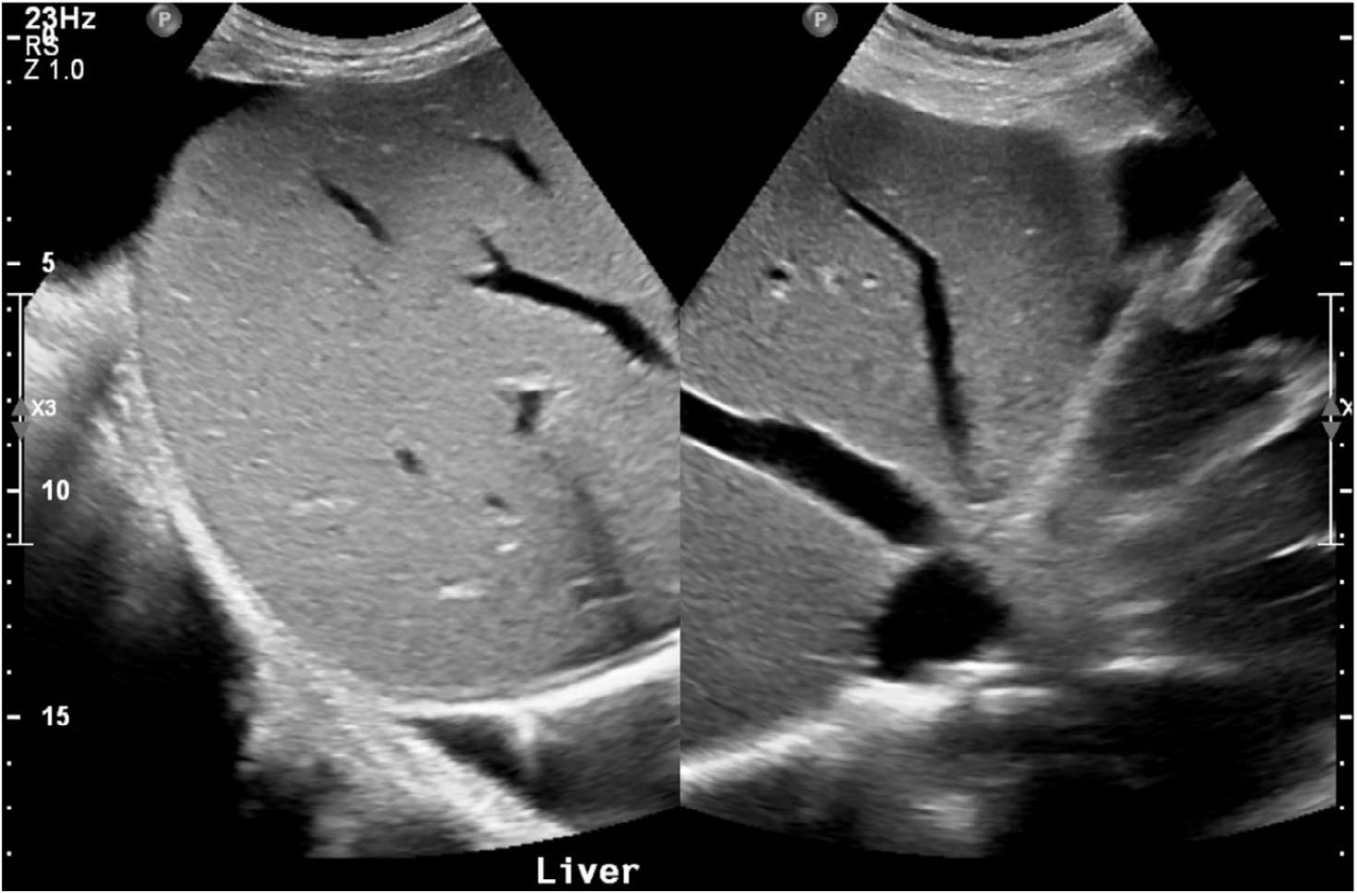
An ultrasound of the abdomen showing congestive hepatopathy with dilated hepatic veins and inferior vena cava.
